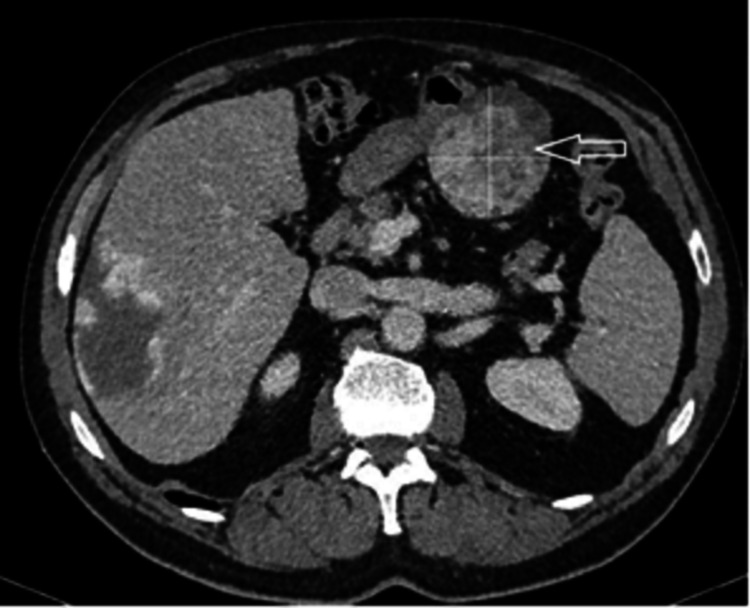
Case 2. Contrast-enhanced abdominal CT scan This shows a heterogeneous mass on the posterior wall of the antrum, part of the stomach (the white cross inside the tumor is to show the borders of the tumor because it is heterogeneous).
